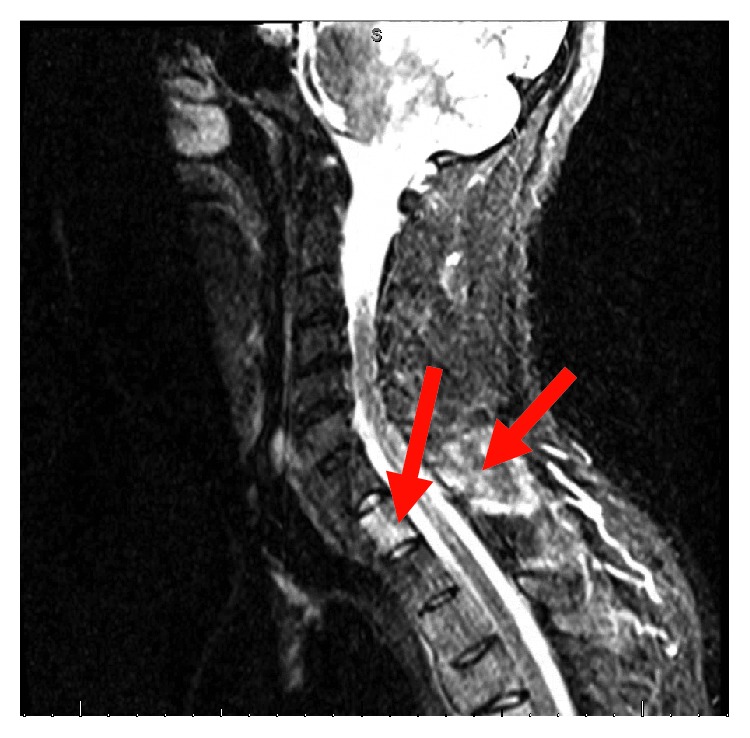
T2 sagittal MRI cervical and lumbar spine.
